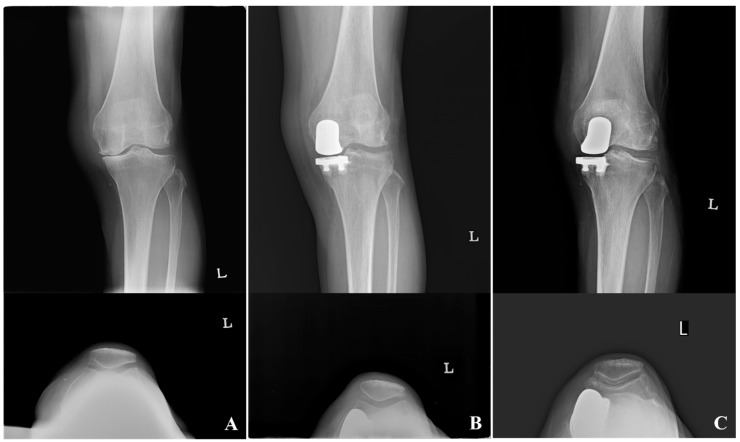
Excellent clinical outcome despite radiographic osteoarthritis progression at a 17-year follow-up after medial fixed-bearing unicompartmental knee arthroplasty (UKA). A 54-year-old female patient who underwent medial fixed-bearing UKA in the left knee. (A) Preoperative knee anteroposterior and merchant radiographs showing medial osteoarthritis. (B) Postoperative knee anteroposterior and merchant radiographs after medial fixed-bearing UKA. (C) Knee anteroposterior and merchant radiographs at 17 years postoperatively demonstrating osteoarthritis progression in the lateral tibiofemoral and patellofemoral compartments. Despite radiographic deterioration, the patient showed excellent clinical outcomes with a WOMAC score of 7.
